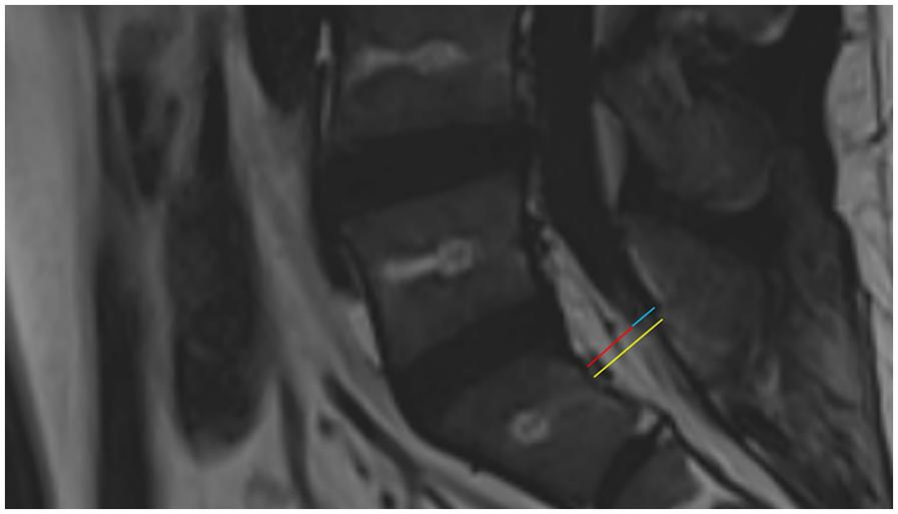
Sagittal T2 FLAIR MRI of the patient. The yellow line shows the anteroposterior maximum diameter of the spinal canal, the red line shows the anteroposterior maximum diameter of the epidural fat, and the blue line shows the anteroposterior maximum diameter of the dural sac.
